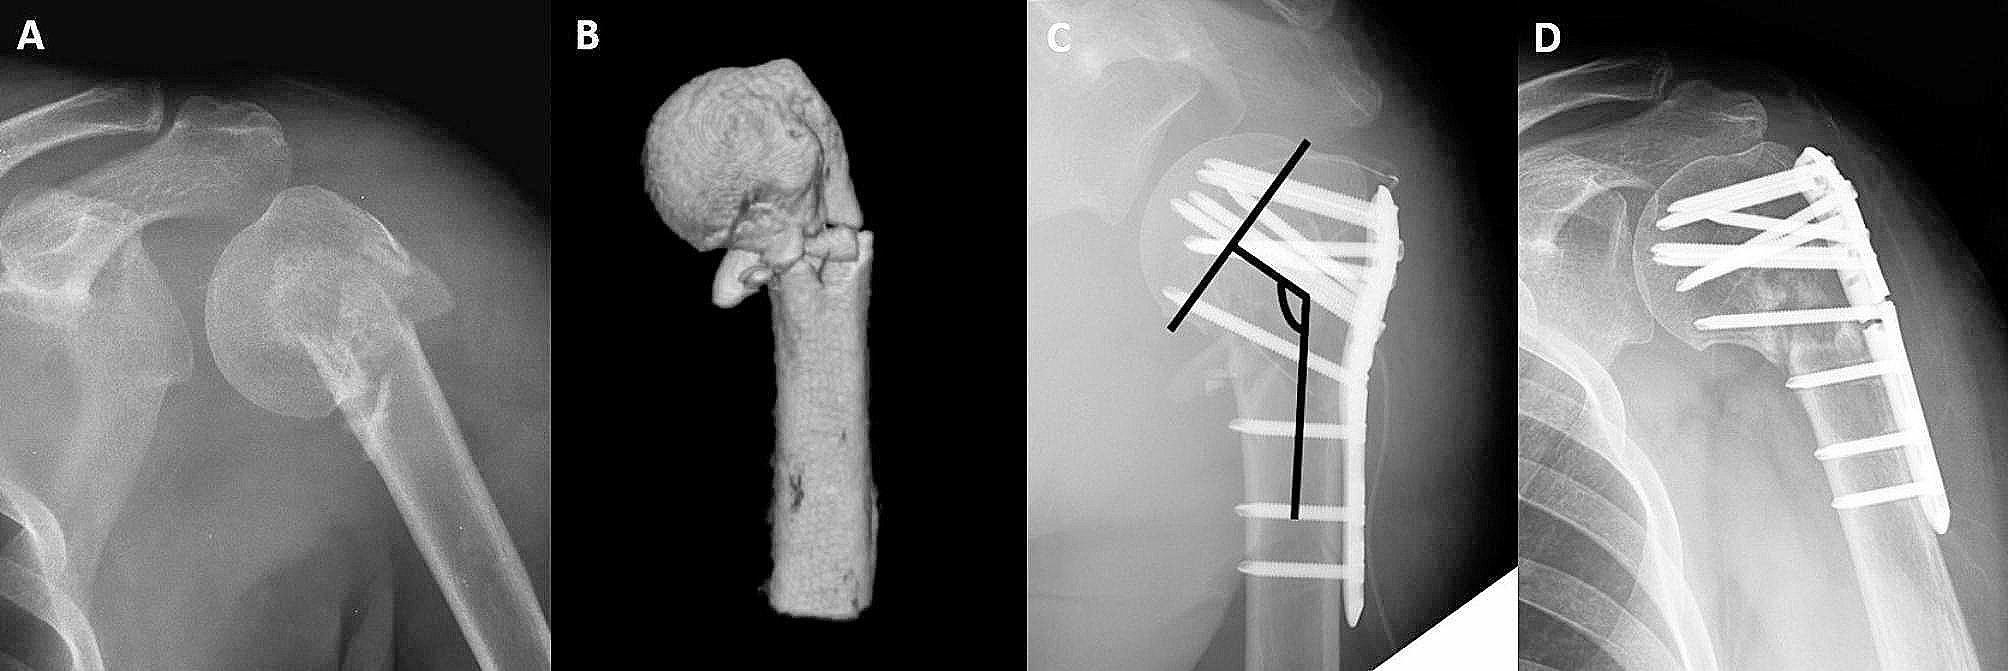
A case demonstrating implant failure, a major complication. A 62-year-old female patient woman sustained a left shoulder injury from slipping down. The shoulder plain radiograph shows a left proximal humerus fracture (PHF), classified as a Neer two-part fracture. (A) Shoulder AP and (B) shoulder 3D CT show medial comminution. (C) Immediate postoperative shoulder AP view shows that one medial supporting screw (MSS) is used, and the immediate postoperative neck–shaft angle (NSA) is 121º. (D) At 1 year postoperatively, implant breakage is observed
